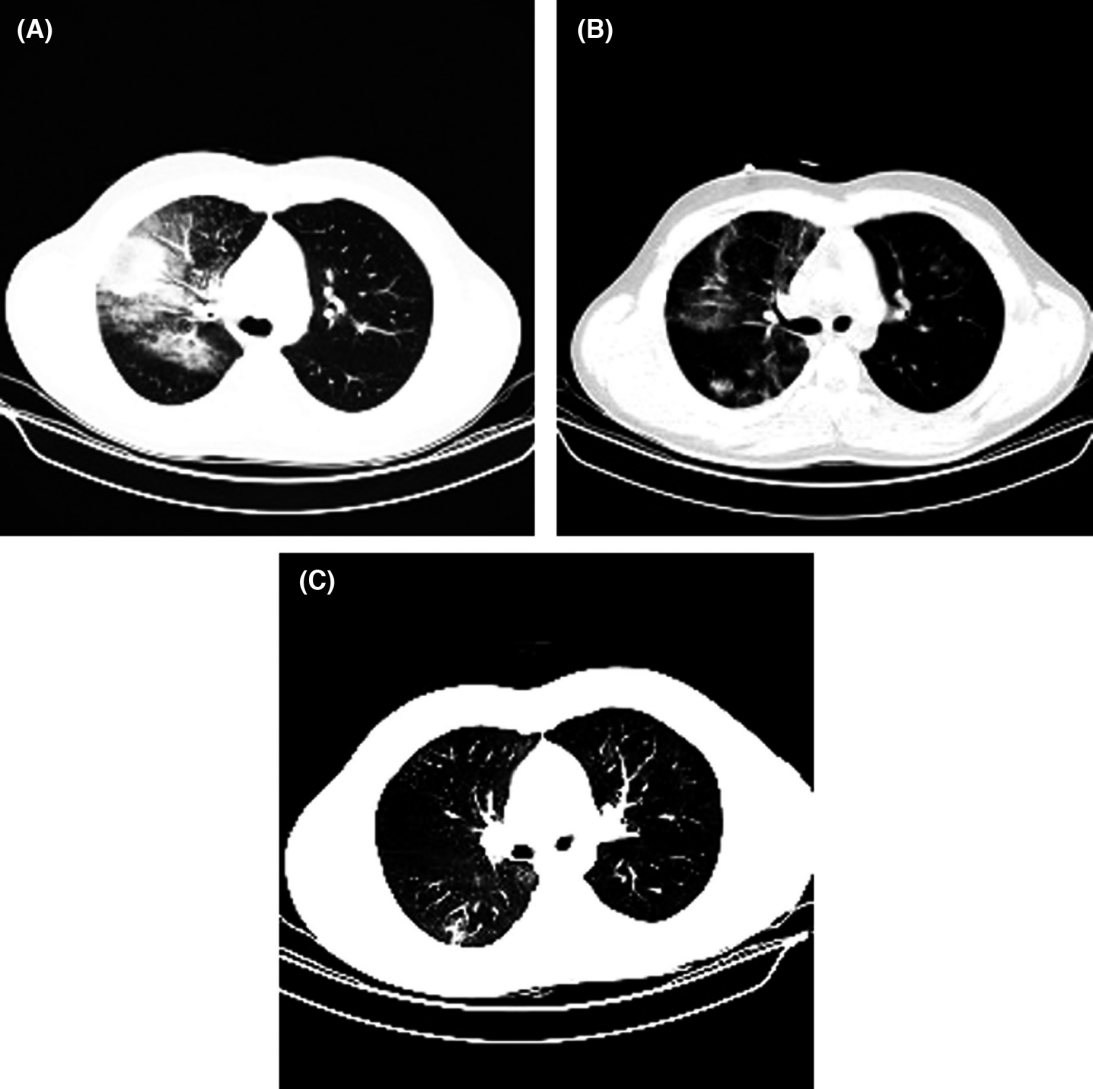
CT scan images (A) The CT result on admission day (B) The CT result 24 h after the first injection of decidual stromal cell administration (C) The CT result 24 h following the second injection of decidual stromal cells
